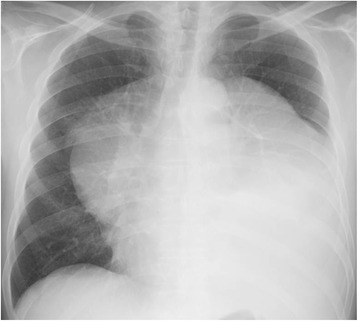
Chest X-ray film showing a huge mediastinal tumor
